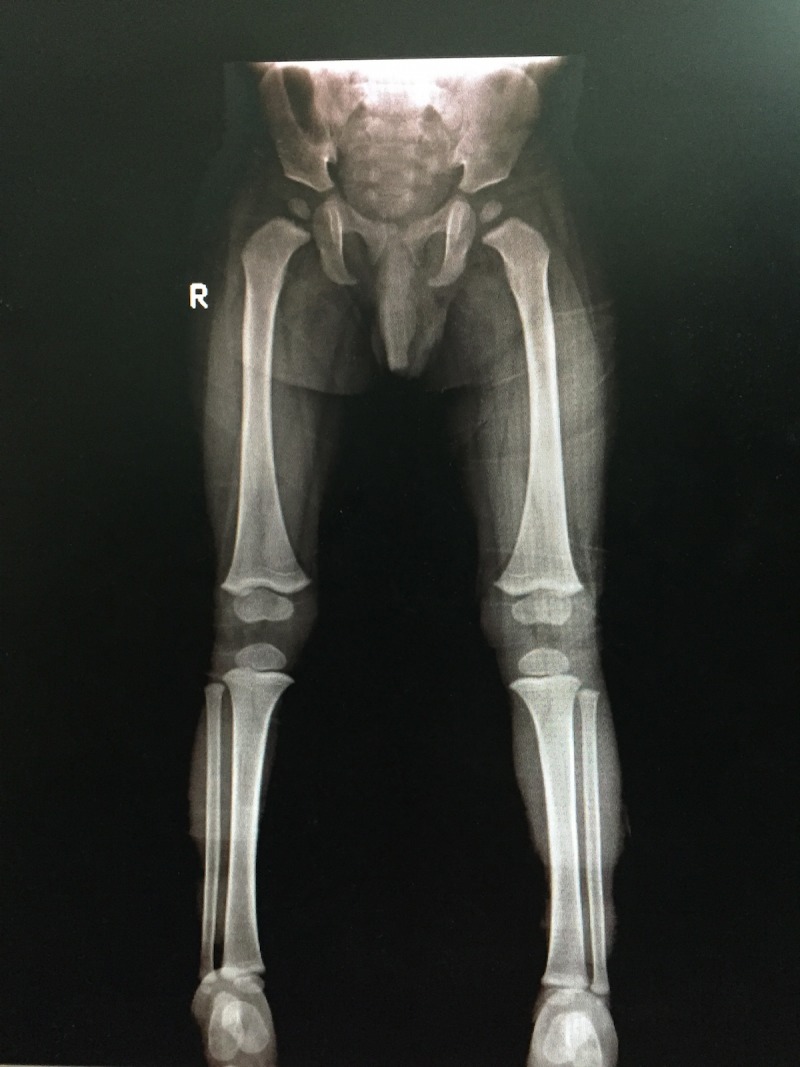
Anteroposterior (AP) view of bilateral lower limbs Follow-up radiograph of the same child after 2.5 years shows improvement with complete resolution of lamellated periosteal bone formation in previously involved left femur and bilateral tibia.
